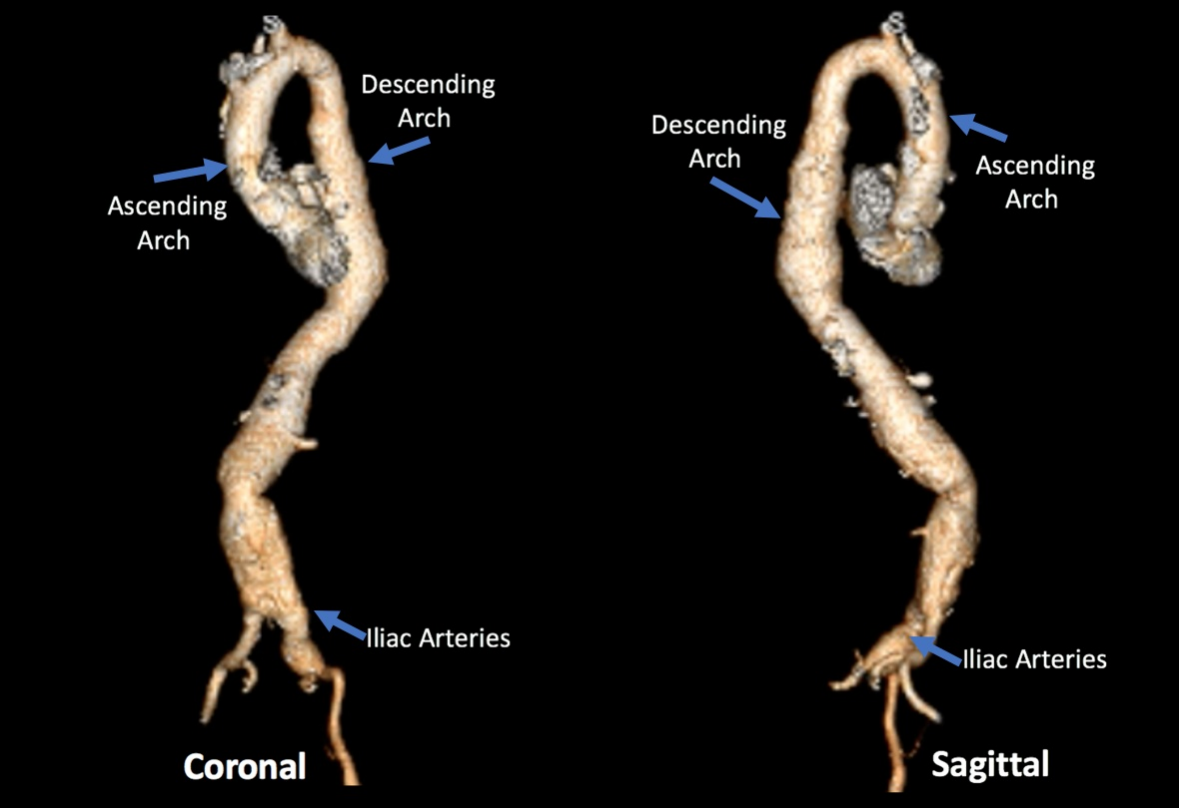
3-dimension aorta. Computed tomography of the aorta with 3-dimmensional reconstruction. Coronal and sagittal imaging of the thoracic and abdominal aorta.
